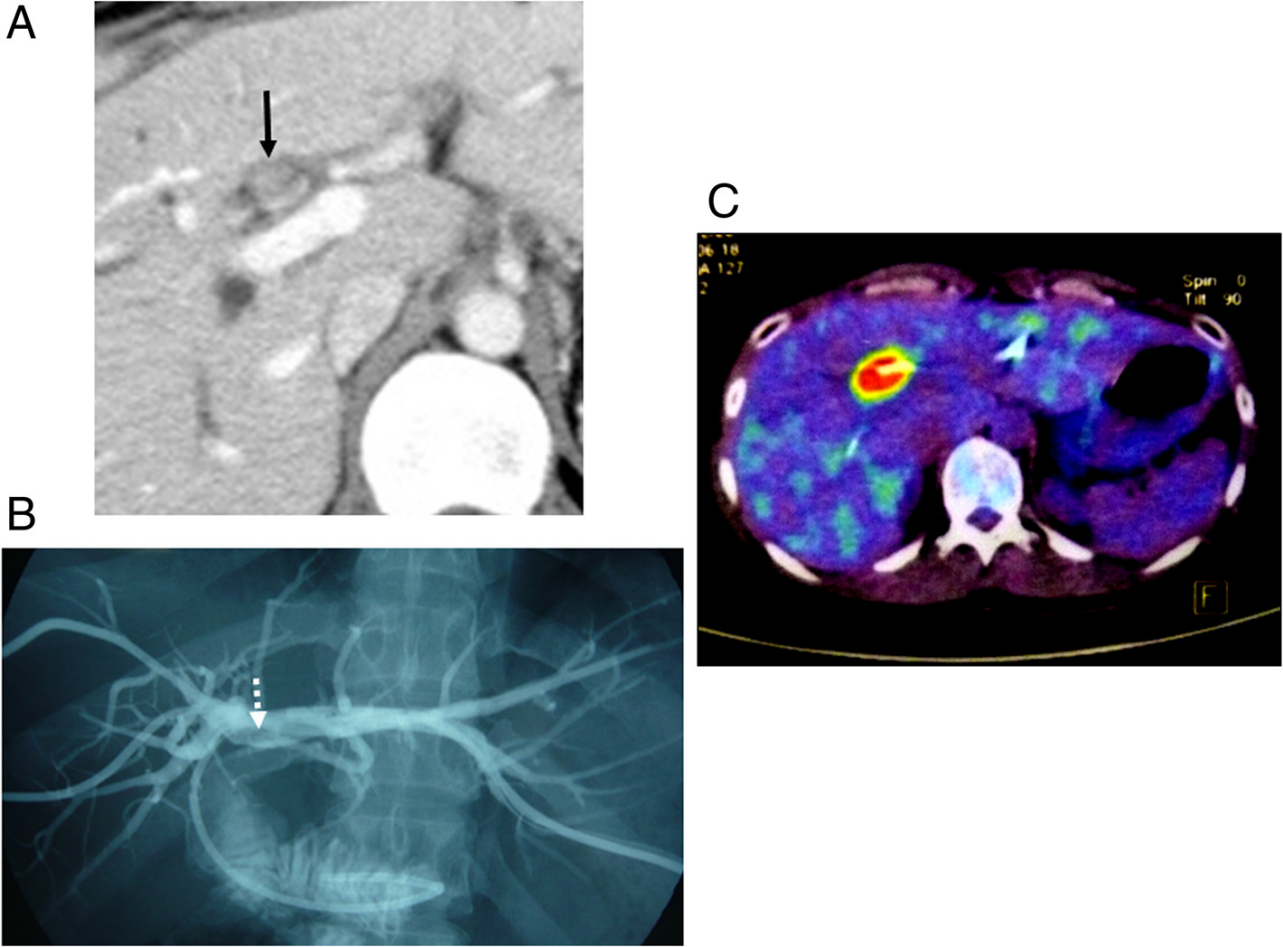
Preoperative images of the third operation. (A) Computed tomography demonstrated wall thickening with mild enhancement of the bile duct at the hepatic bifurcation adjacent to the jejunum (arrow) and upstream dilatation of the bilateral hepatic ducts. (B) Catheter cholangiogram through the percutaneous transhepatic biliary drainage tube revealed a filling defect at the site of hepaticojejunostomy (dotted arrow). (C) Positron-emission tomography revealed an abnormal uptake at the anastomotic site.
